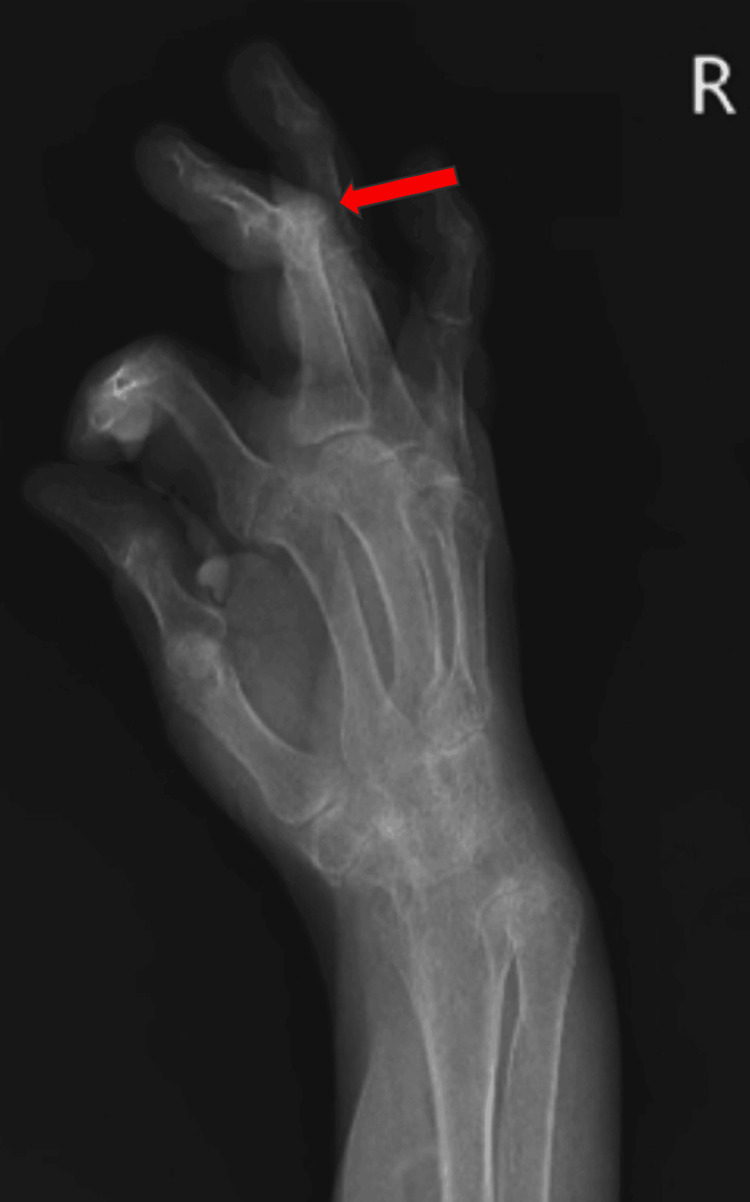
Radiograph showing the interphalangeal joint
